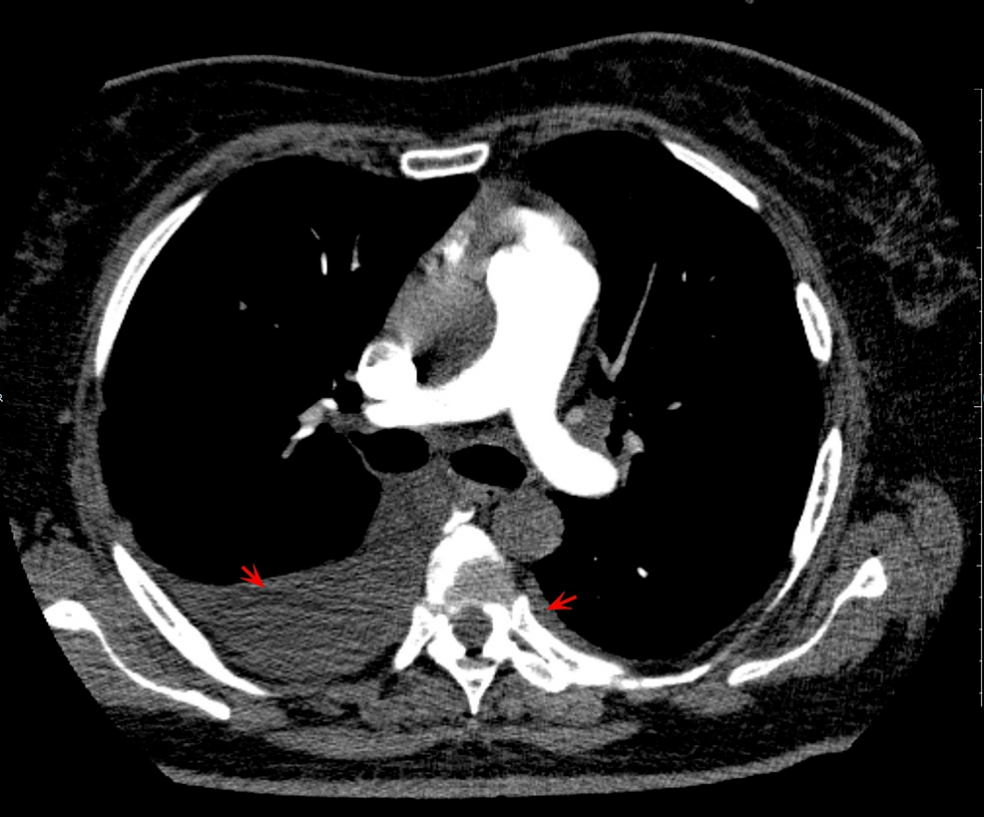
CT pulmonary angiogram showing bilateral pleural effusion (arrows), larger in the right hemithorax.
